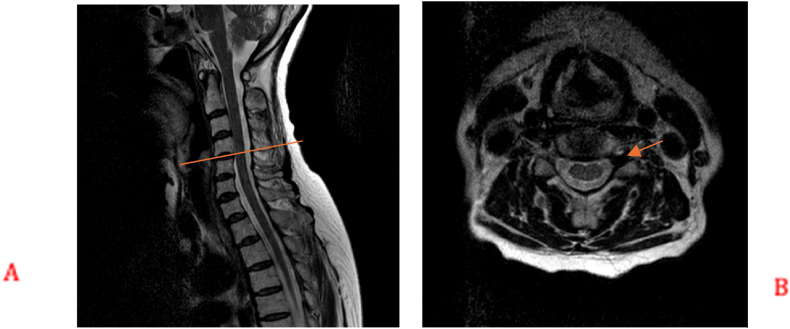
(A) MRI spine sagittal T2 did not reveal overt disc degeneration. (B) MRI spine axial T2 showing abnormal ectatic artery in the left C4-5 foramen.
